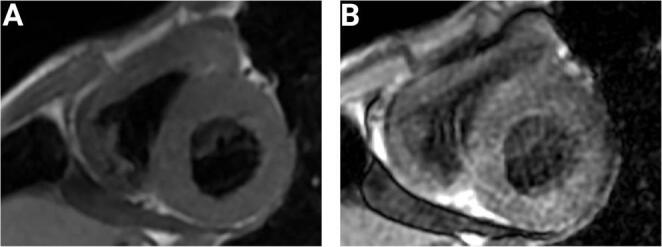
Cardiovascular magnetic resonance imaging of a patient with cardiac amyloidosis. Panel A: Pre-contrast image. Panel B: Late gadolinium enhancement (LGE) image
